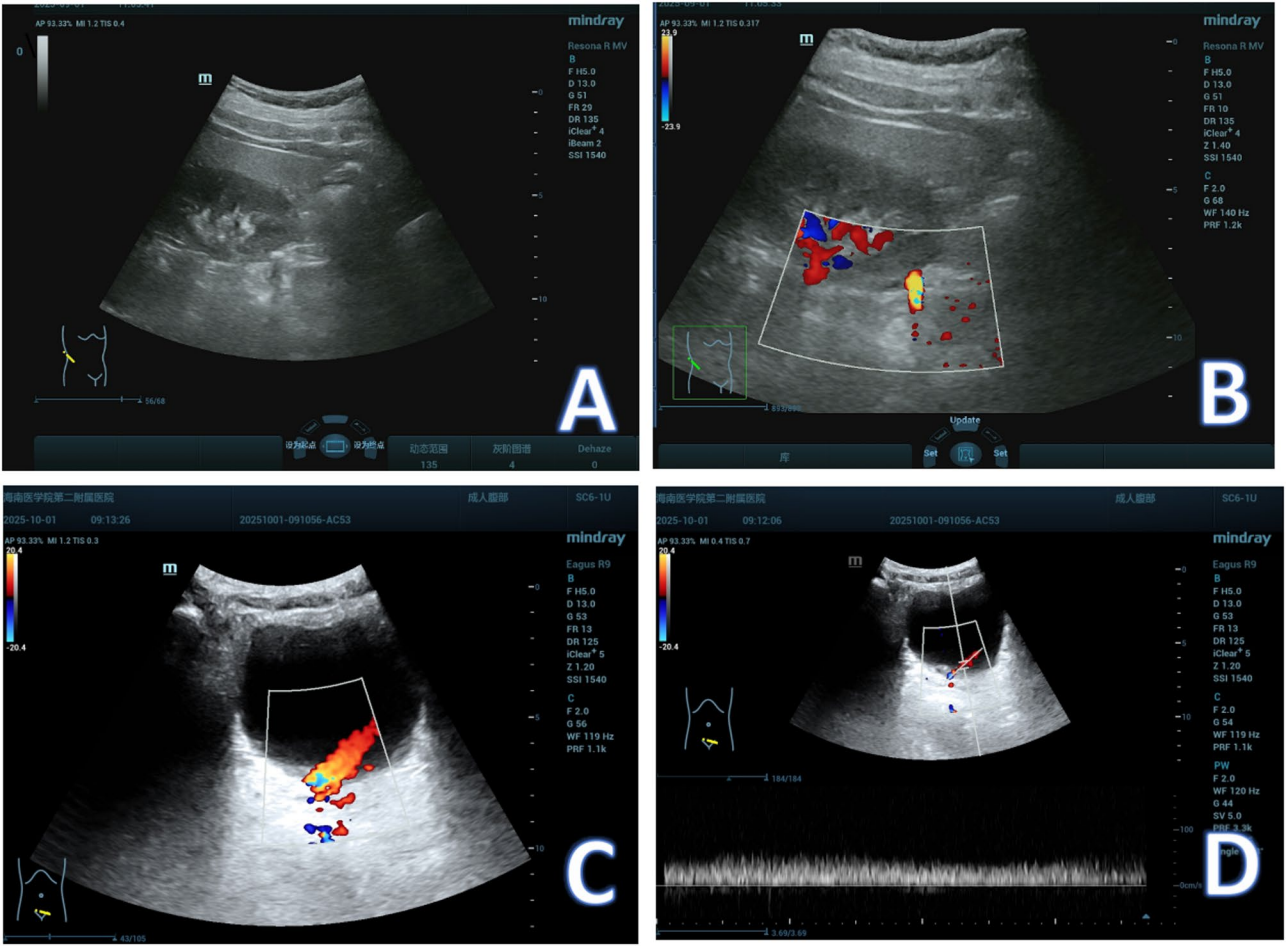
Male, 18 years old, with right upper ureteral stone. (A)longitudinal view of ureteral stone, (B) twinkle artifacts of ureteral stone, (C) Color Doppler image of ureteral squirt, and (D) spectral Doppler image of ureteral jet (jet frequency: 2.0 times/min)
